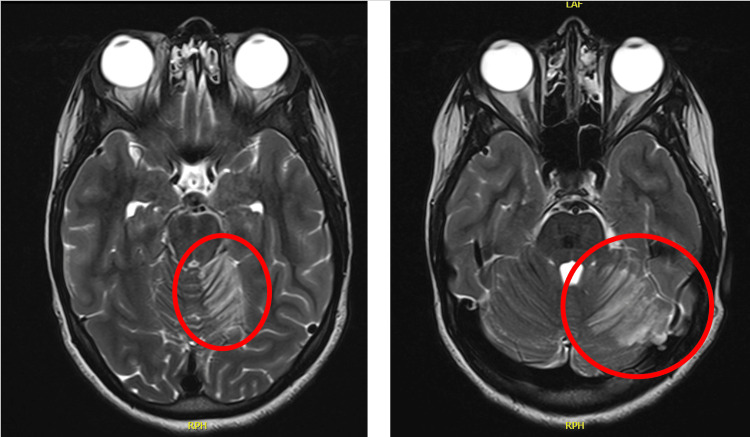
T2-weighted, turbo spin echo MRI of the brain showed T2 hyperintensity in the superolateral aspect of the left cerebellar hemisphere and left medial temporal lobe, suggestive of a non-hemorrhagic infarct in the left superior cerebellar artery territory.
